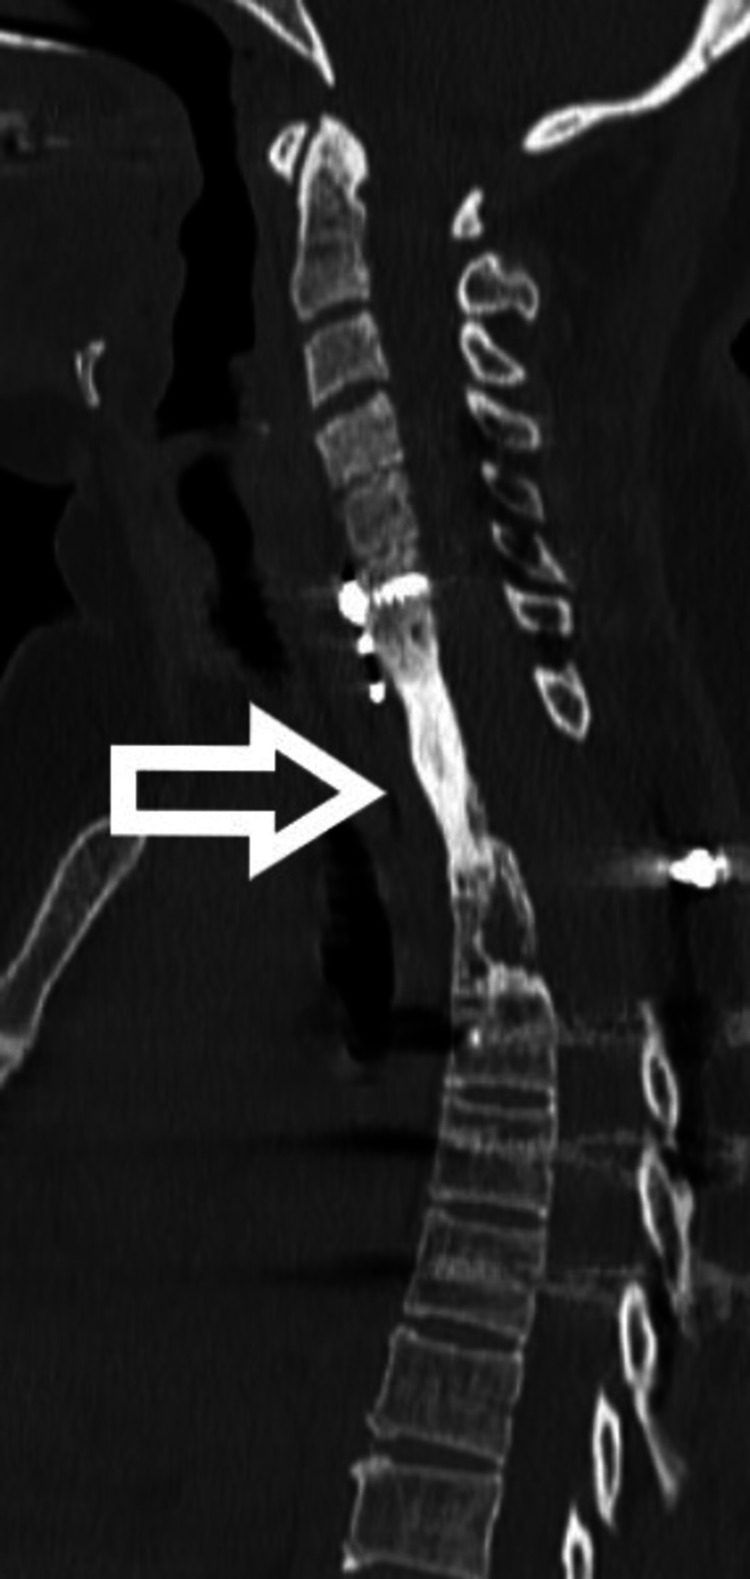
CT scan shows solid incorporation of fibular graft
